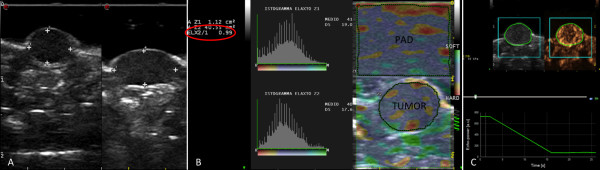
B-mode, elastonosonography and contrast enhanced ultrasonography with VEGFR2 targeted microbubbles images. B-mode ultrasonography (panel A) was used to calculate tumor dimensions whereas elastosonography (panel B) allowed to evaluate the elasticity of the tumor with respect to a pad with costant elasticity. Elasticity ratio is reported (ELX2/1) as well as histogram of elasticity distribution. In panel C, contrast enhanced ultrasonography with VEGFR2 targeted microbubbles images immediately before the high mechanical index flash (5 minutes and 59 seconds) is shown (upper part of the panel). Time intensity curve (lower part of panel C) was created using a dedicated software to calculate differential targeted enhancement (dTE).
